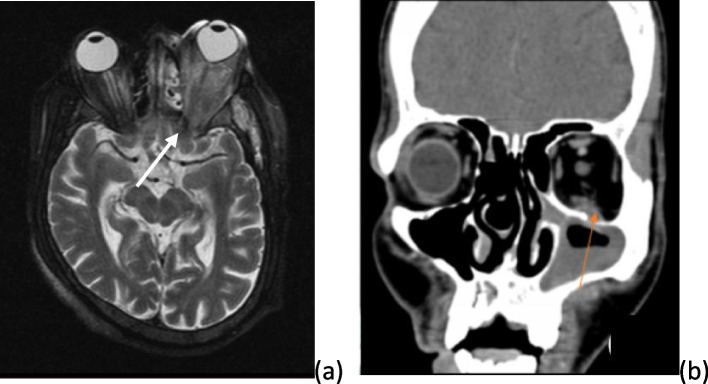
a, b Radiographic pictures of case series 3. a Thickened superior ophthalmic vein with possible cavernous sinus thrombosis on MRI. b CT image showing opacities in (L) maxillary sinus extending into (L) orbit through a breach in the orbital flo
